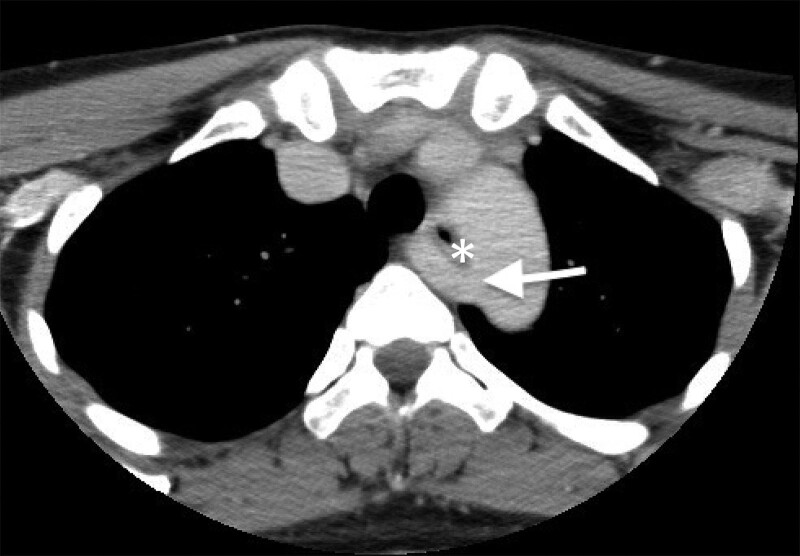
Contrast-enhanced computed tomography reveals an aberrant right subclavian artery (arrow) arising from the left aortic arch, which is located posterior to the esophagus (asterisk), suggesting a retroesophageal course.
